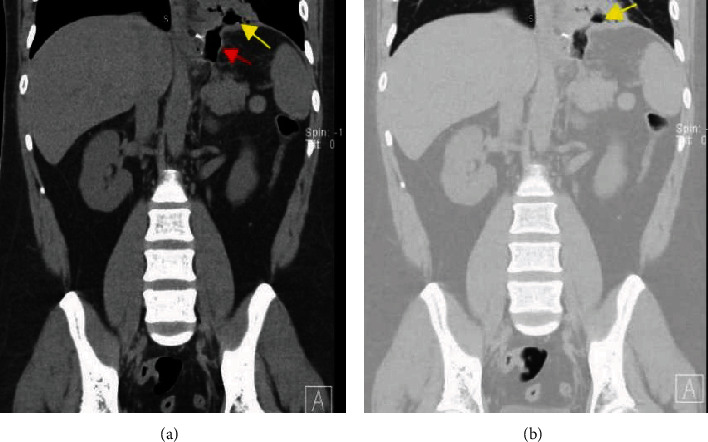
Coronal nonenhanced CT image of the lower chest and upper abdomen (soft tissue and lung window (a, b)) shows left lower lobe consolidation with cavitation (yellow arrow), communicating with the lumen of the gastric remnant (red arrow).
